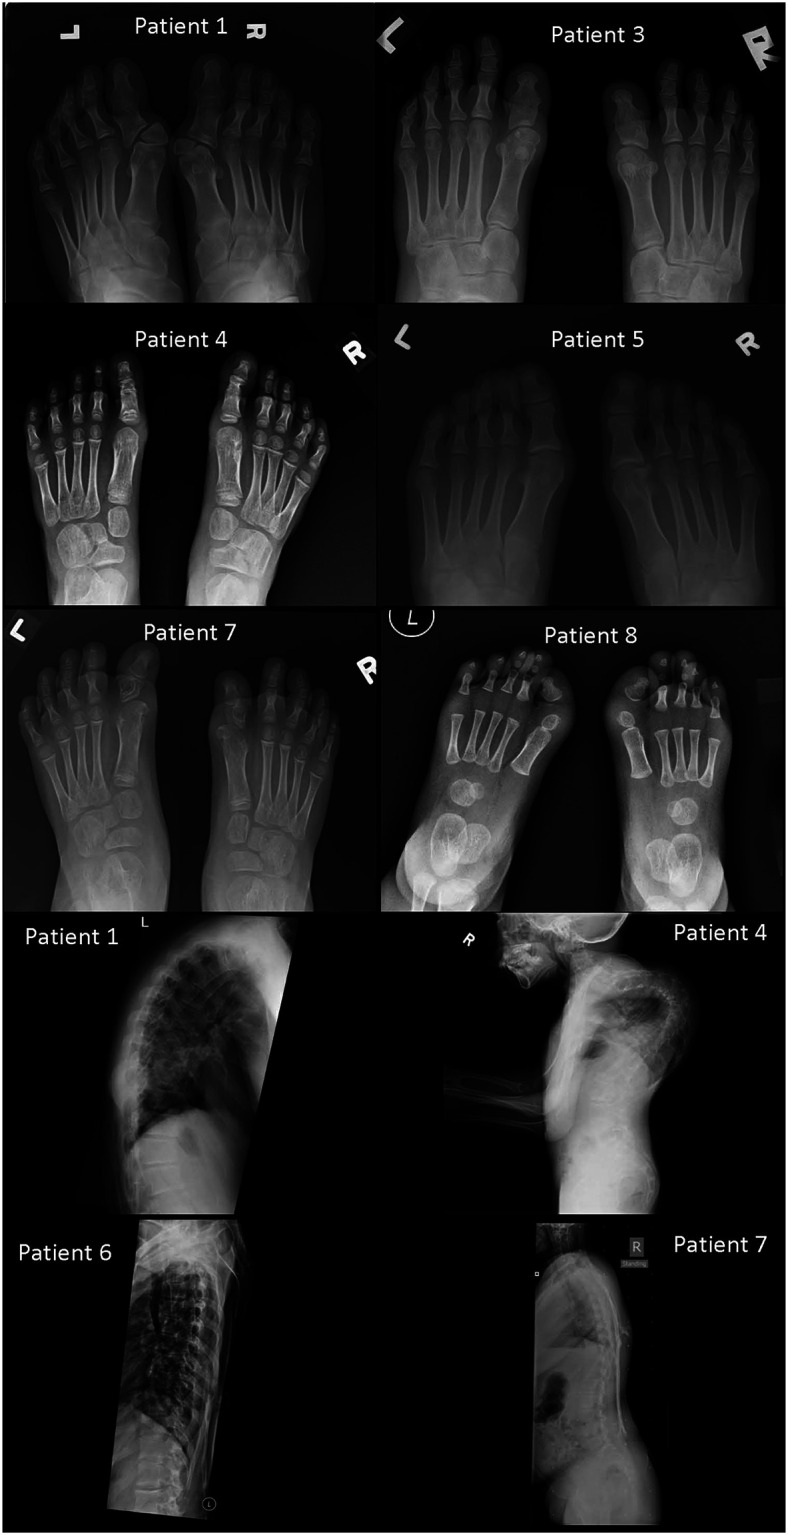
Foot radiographs from Patients 1, 3, 4, 5, 7, and 8, and spine radiographs from Patients 1, 4, 6, and 7 (foot radiographs). Patient 1: Deformed heads of bilateral first metatarsals and proximal phalanges with hallux valgus. Fused proximal and distal phalanges of both big toes. Patient 3: Deformed heads of bilateral first metatarsals and phalanges of bilateral big toes. They are hypoplastic and short with fusion of proximal and distal phalanges of both big toes. Patient 4: Tri-phalangeal big toes on both sides with fusion of the proximal and middle phalanges. Subtle hallux valgus. Patient 5: Subtle hallux valgus. Patient 7: Dysplastic heads of bilateral first metatarsals and proximal phalanges of both big toes. Right big toe appears short. Patient 8: Bilateral heads of first metatarsals appear dysplastic with short hypoplastic bilateral big toes with single phalanx. Bilateral hallux valgus (spine radiographs) Patient 1: Ossifications of the paravertebral soft tissue demonstrated on the lateral view with a kyphotic spinal curvature. Patient 4: Extreme kyphoscoliosis of the whole spine with segments of cervical spine fusion and impending chin-on-chest deformity. Patient 6: Relative preservation of normal thoracic spinal alignment with no major heterotopic ossifications. This spinal radiograph is taken at similar age as that of Patient 1. Patient 7: Paraspinal soft tissue ossifications extending from upper back down to upper sacral level with relatively preserved vertical spinal alignment. This spinal radiograph is taken at similar age as that of Patient 4.
